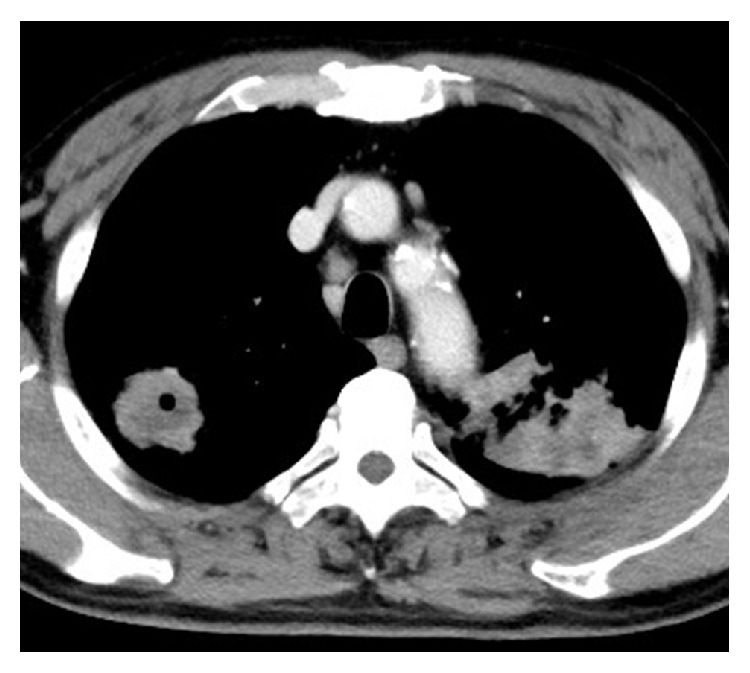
Chest CT (mediastinal window) scan showed bilateral pulmonary nodules and a solid, enhancing mass with cavitation of 6.5 cm at its largest diameter.
